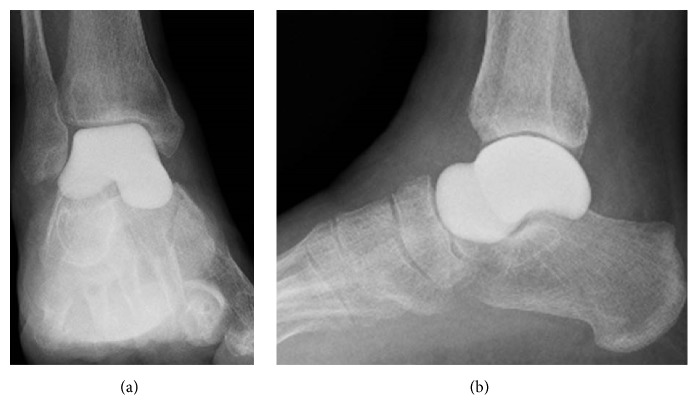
Postoperative radiographs showing replacement of the talus with an alumina ceramic total talar prosthesis in anteroposterior (a) and lateral (b) views at 1 year and 6 months after surgery. There is excellent congruence between the talar prosthesis and the tibia, fibula, calcaneus, and navicular bone.
